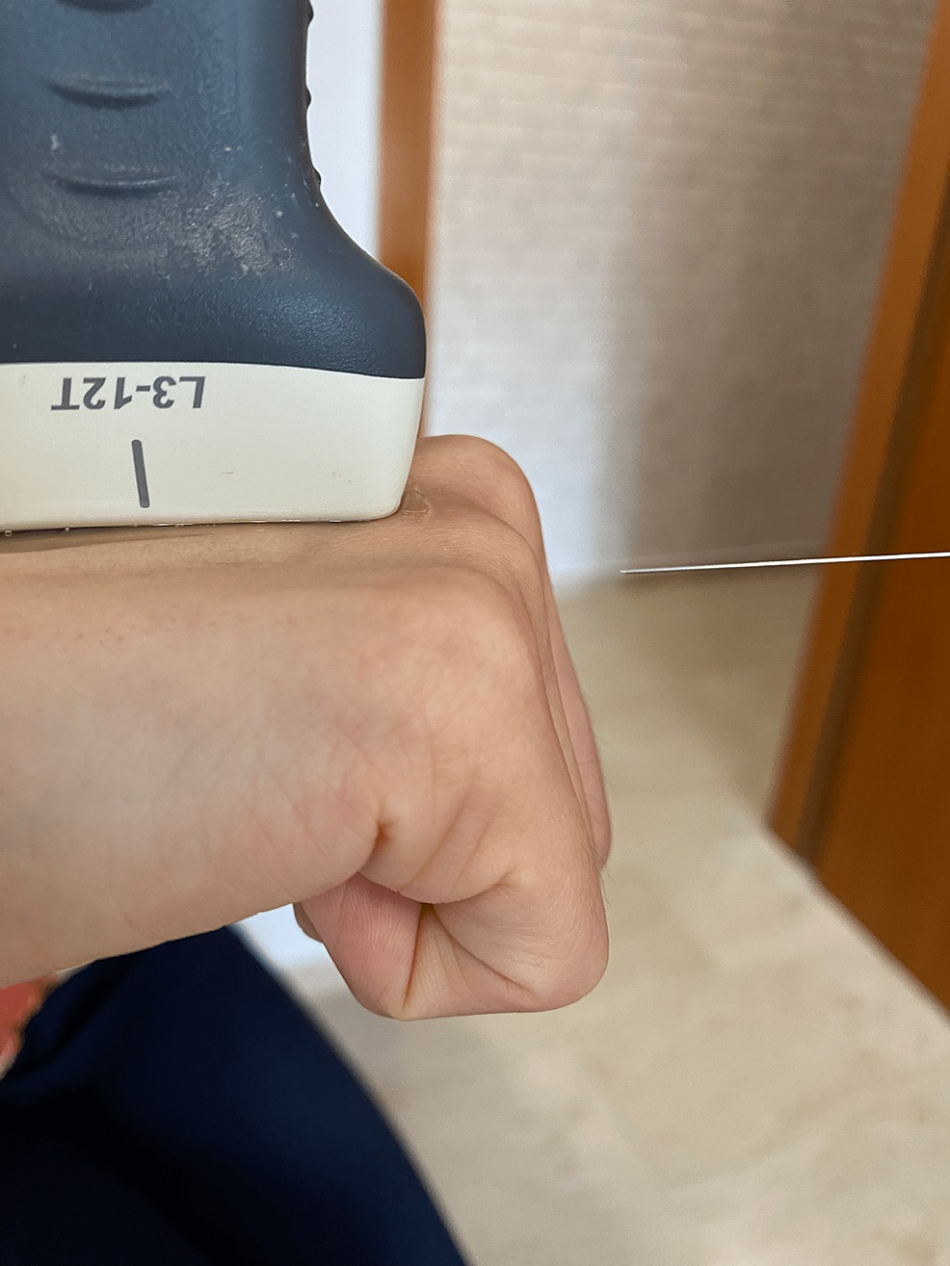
In-lane US-guided injection. Insertion point of the needle. This is the insertion point for the needle in an in-plane ultrasound-guided injection technique for a patient with upper limb spasticity [6]. The insertion point was selected to ensure a parallel ultrasound view of the needle.
